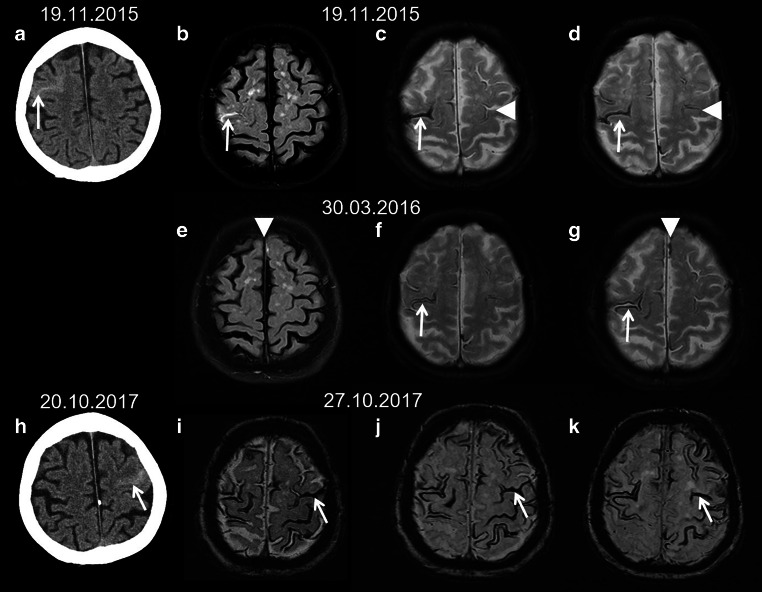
A 74-year-old woman suffering from recurrent cortical subarachnoid hemorrhage (cSAH) in cerebral amyloid angiopathy (CAA). a–d First cSAH frontal right (a: CT ax., *arrow*) with sulcal hyperintense signal changes on fluid attenuated inversion recovery (FLAIR) images (b, *arrow*) and sulcal signal loss on T2*WI (c,d: *arrow*); additional cortical superficial siderosis (cSS) left (c,d: *arrowhead*); e–g second cSAH paramedian frontal left (e: FLAIR ax.; g: T2*WI ax.; *arrowhead*); note characteristic bilinear track-line appearance of cSS in the chronic stage (f,g: T2*WI, *arrow*); h–k third cSAH frontodorsal left (h: CT ax., *arrow*) with signal loss on SWI (i–k, *arrow*) and progressive cSS bilaterally
